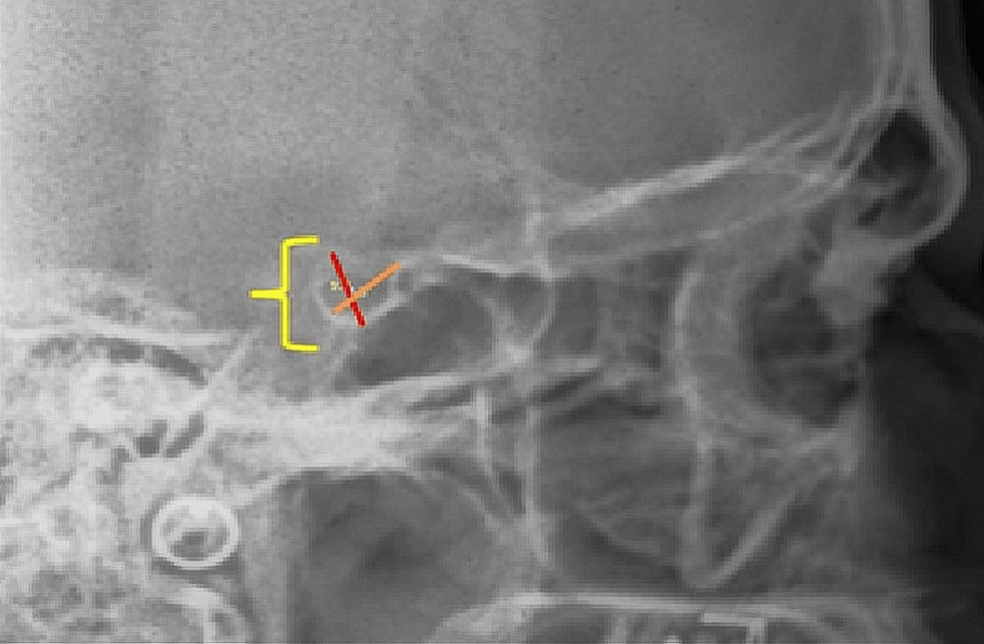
Lateral cephalometric radiograph (with lines marked on the sella; red for depth and orange for length).
